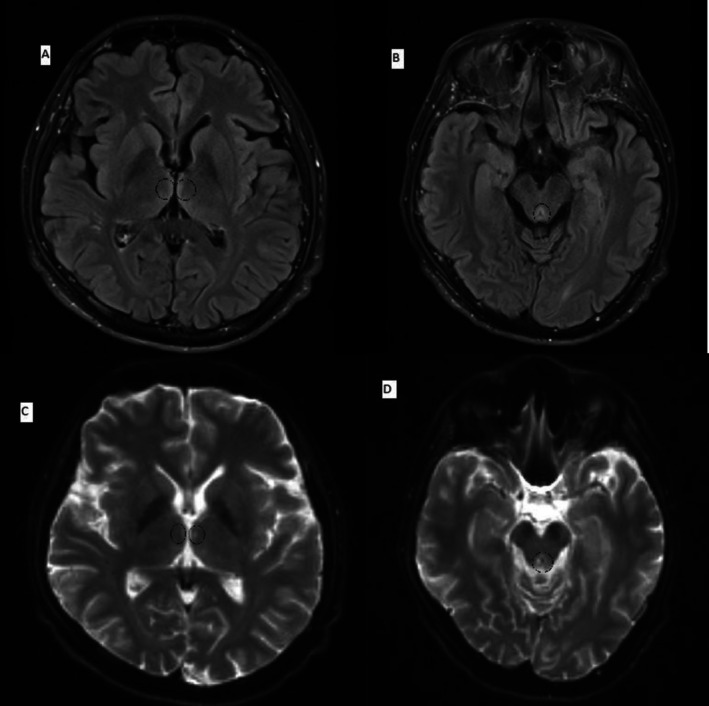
MRI of the brain demonstrating hyperintense lesions in the bilateral medial thalami (A) and periaqueductal region (B). These areas exhibit mild diffusion restriction on the corresponding diffusion‐weighted images (DWI) (C, D).
